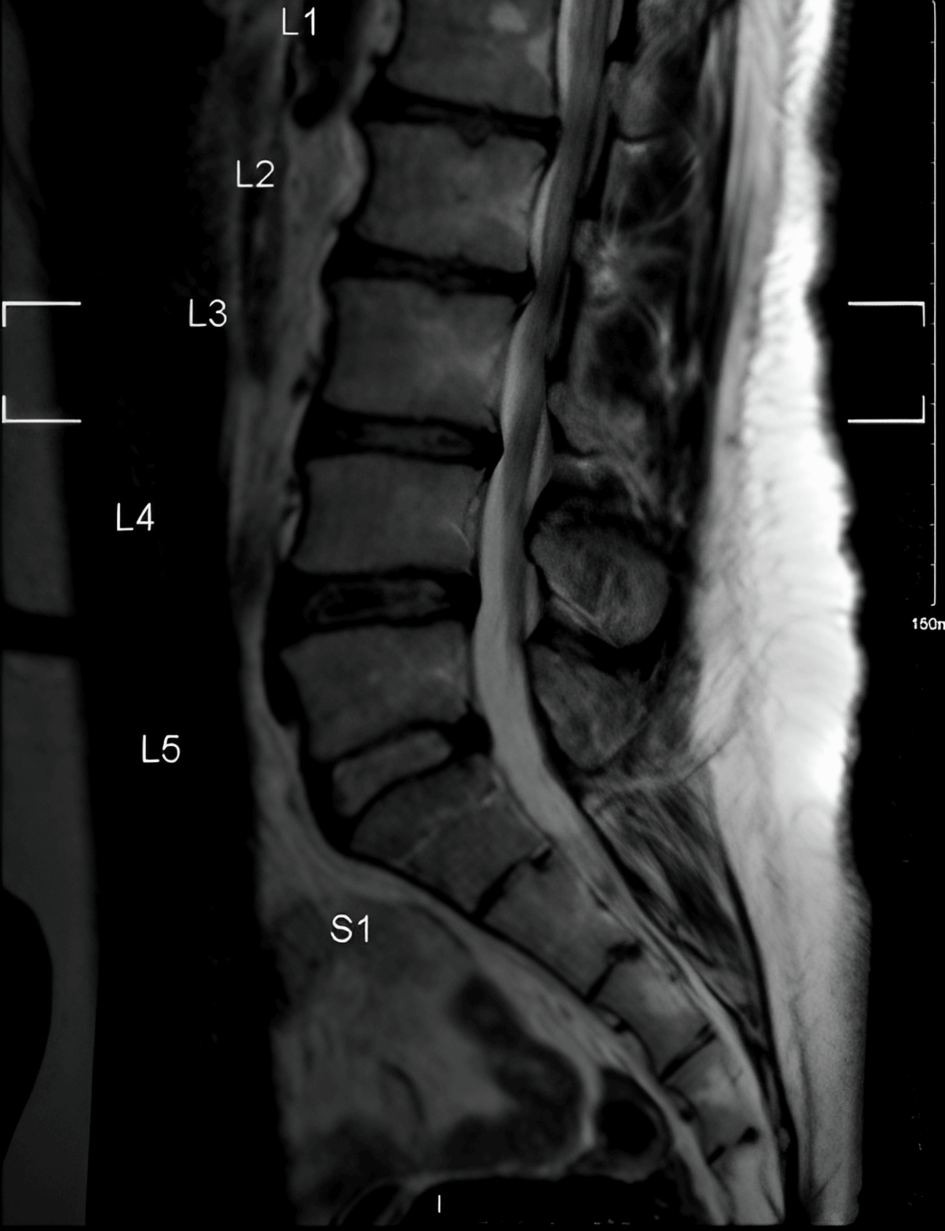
Midline sagittal T2-MRI image showing a normal lower spine segment with mild degenerative changes
